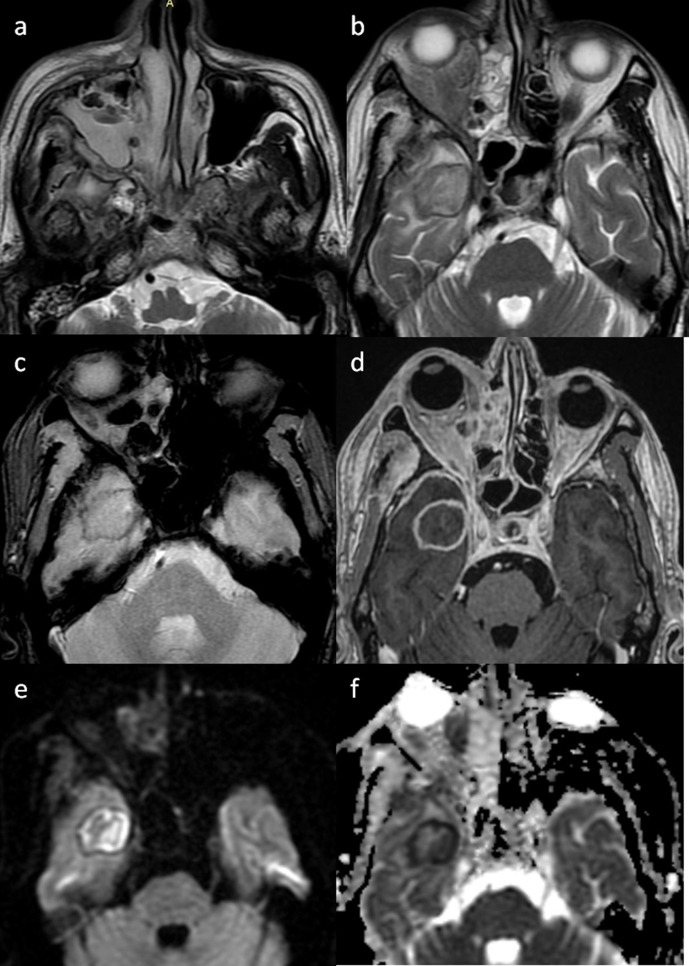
Axial MR images of mucormycosis with intraparenchymal abscess formation. Sinusitis (a) with peripherally hypointense lesion on T2W image (b) showing continuous rim of blooming on SWI (c) and showing rim enhancement on postcontrast T1W image (d). Diffusion sequence images (e, b1000; f, ADC map) show diffusion restriction in the periphery and intracavitary projections with low ADC values.
